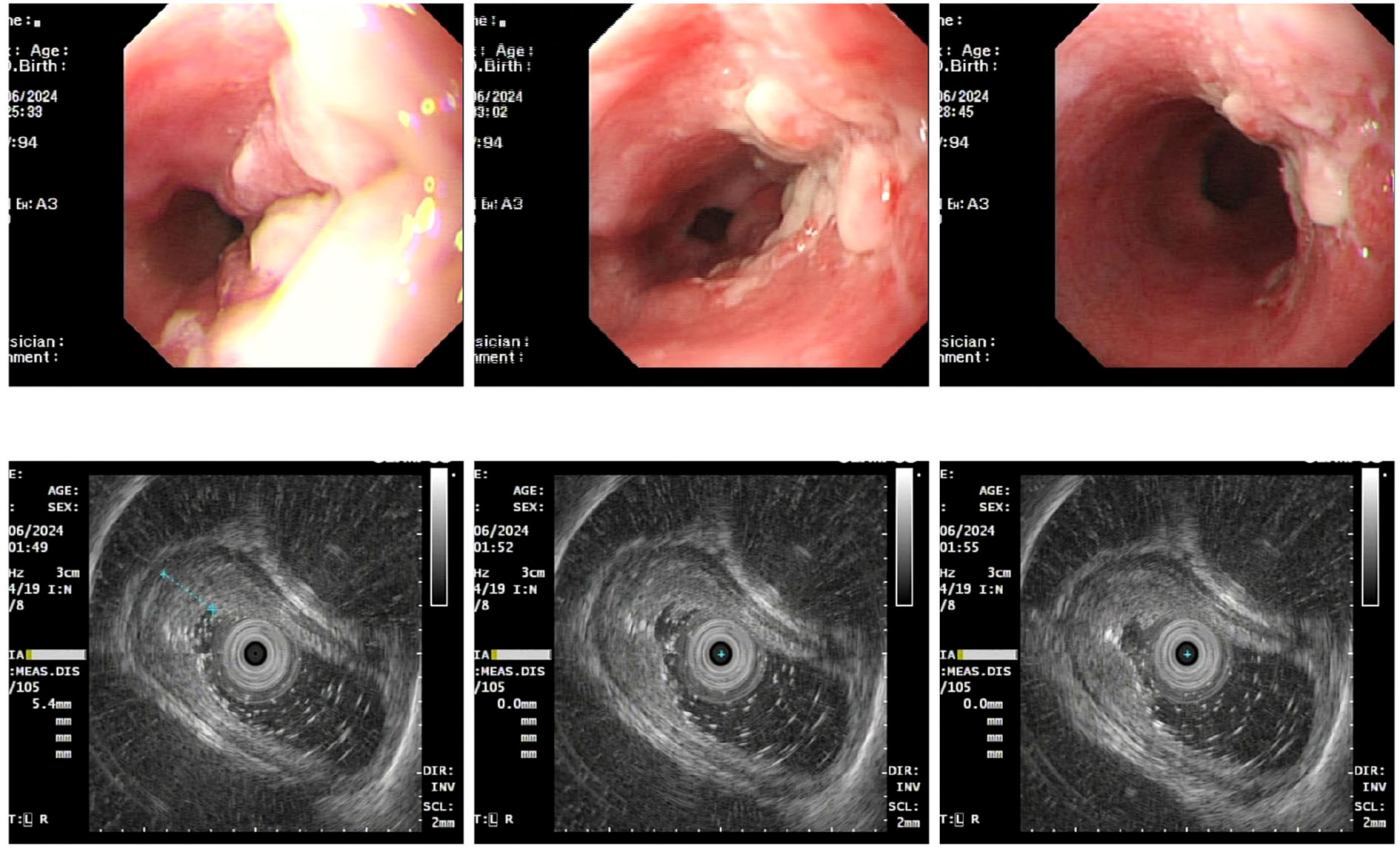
Esophageal lesions visualized under endoscopic ultrasonography.
